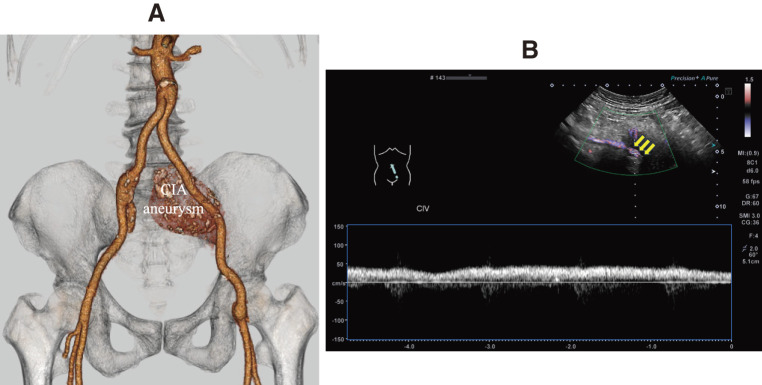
Fig. 3 (A and B) Postoperative computed tomography and abdominal ultrasonography. Three-dimensional computed tomography reveals good revascularization using a bifurcated prosthetic graft, with traces of the left CIAA remaining (A). Abdominal ultrasonography confirms blood flow into the left CIV, which was reconstructed using the aneurysm wall. No significant changes in intravenous flow velocity are observed (yellow arrows) (B). CIAA: common iliac artery aneurysm; CIV: common iliac vein
